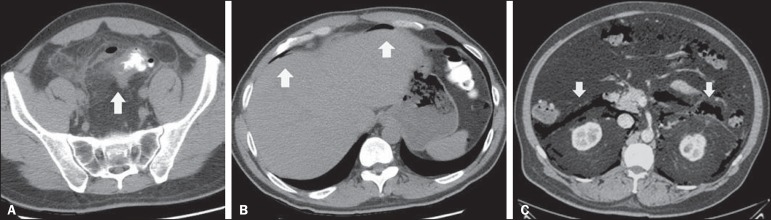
A,B: Rectal contrast-enhanced CT of abdomen showing
